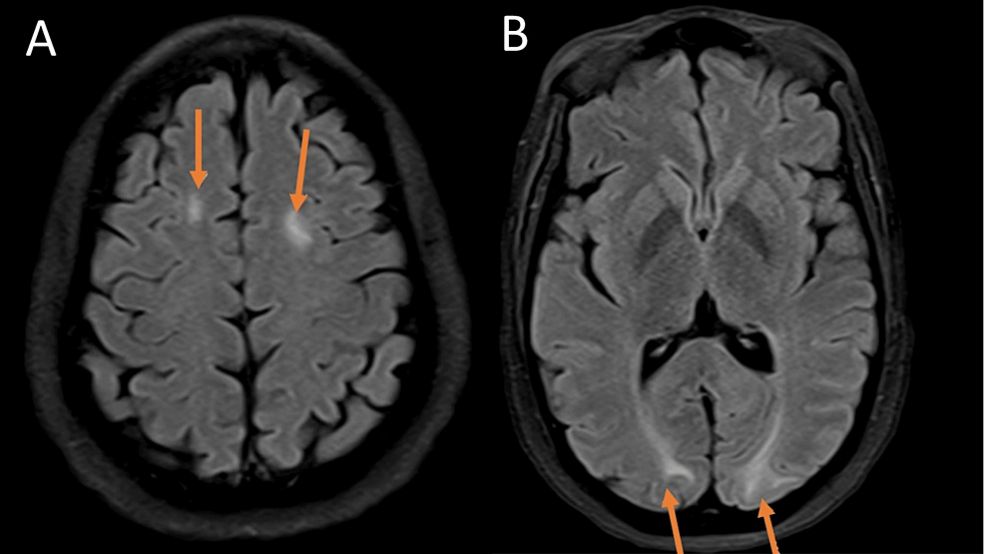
MRI without contrast Figure 1A demonstrates an axial view of the brain using MRI without contrast, demonstrating lupus cerebritis. There were new areas of increased T2/FLAIR signal in the bilateral occipital lobe subcortical white matter, the bilateral parietal periventricular white matter pointed by orange arrows and the bilateral frontal centrum semiovale. Additionally, there is a patchy high flair signal in the pons and a suspected small high T2 signal lesion in the right cerebellum. Figure 1B shows subtle petechial hemorrhages in the bilateral cerebellum and right pons, depicted by orange arrows.
